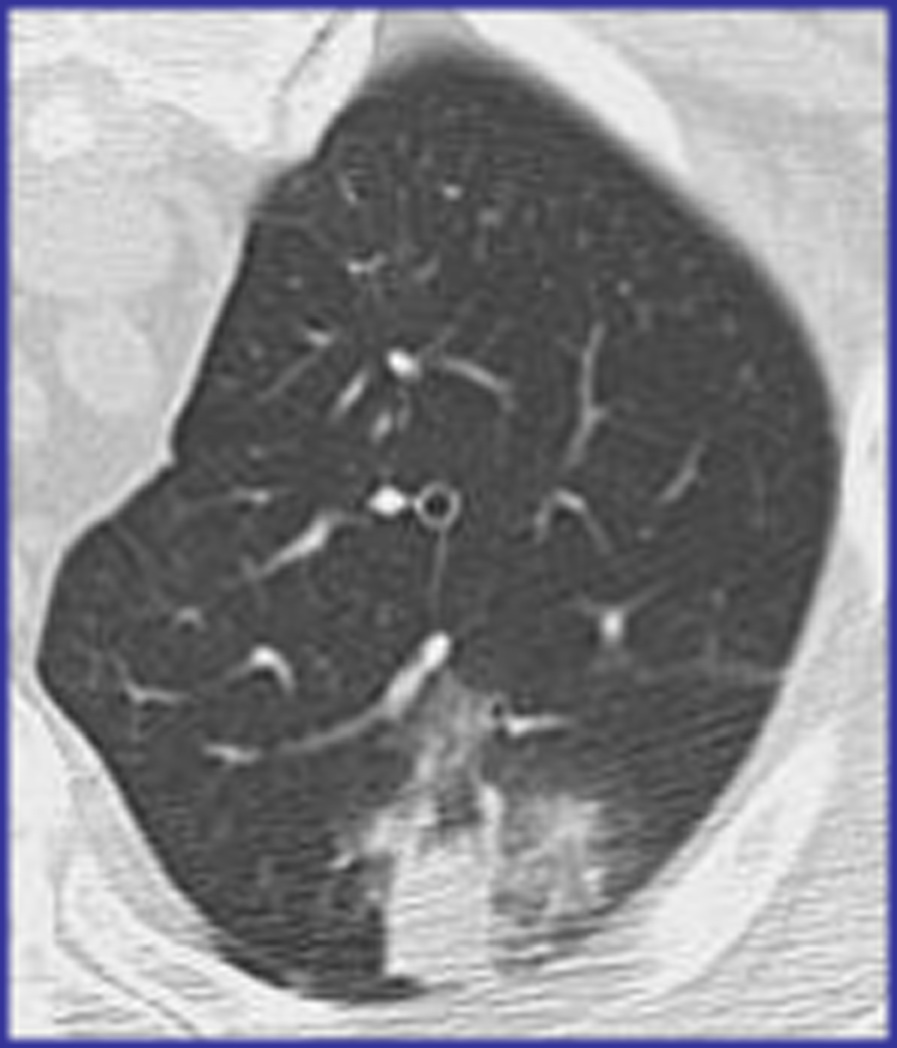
Angioinvasive pulmonary aspergillosis. Patient with chemotherapy for acute myeloid leukaemia. Chest CT at the level of the apical segment of the left upper lobe at lung window: Focal area of consolidation surrounded by a “halo” of ground glass
